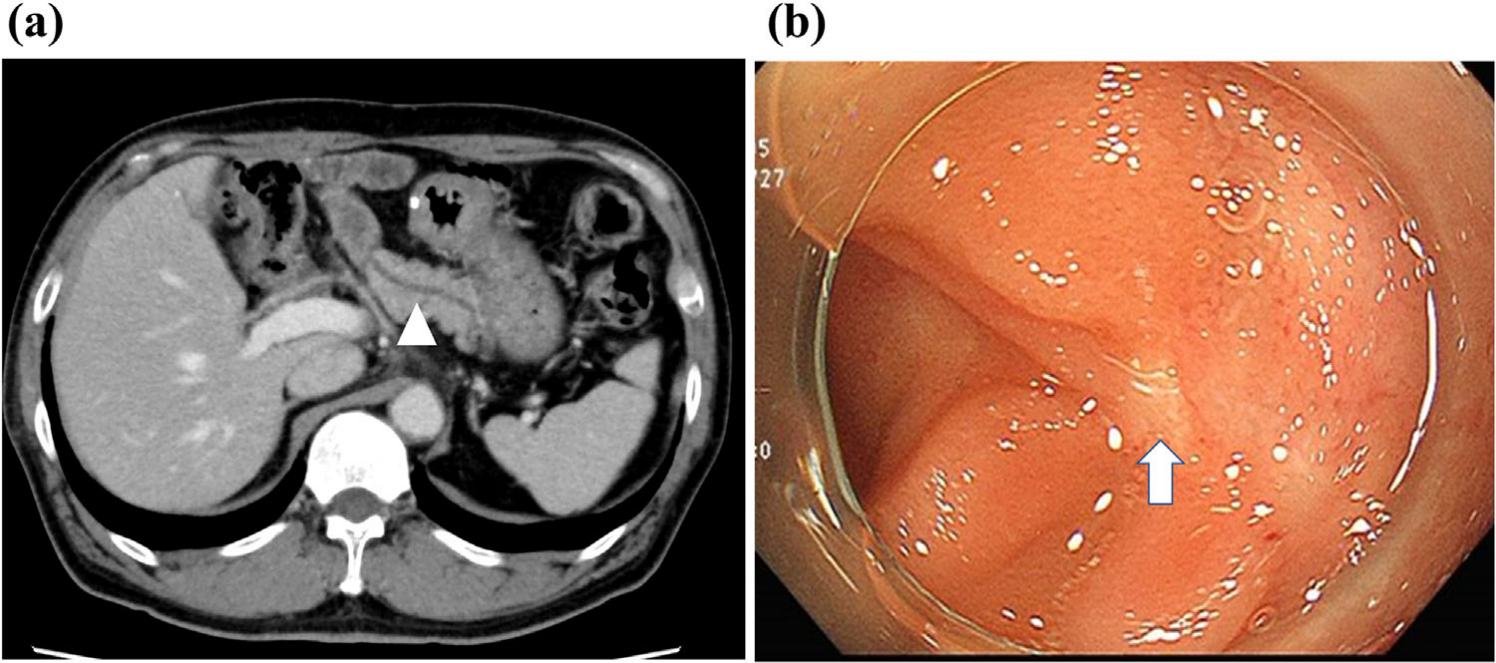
Pretreatment imaging findings. (a) Computed tomography revealed a dilated main pancreatic duct from the anastomosis (arrowhead) in the remnant pancreas. (b) Endoscopic imaging of obstructed pancreatic jejunostomy anastomosis
